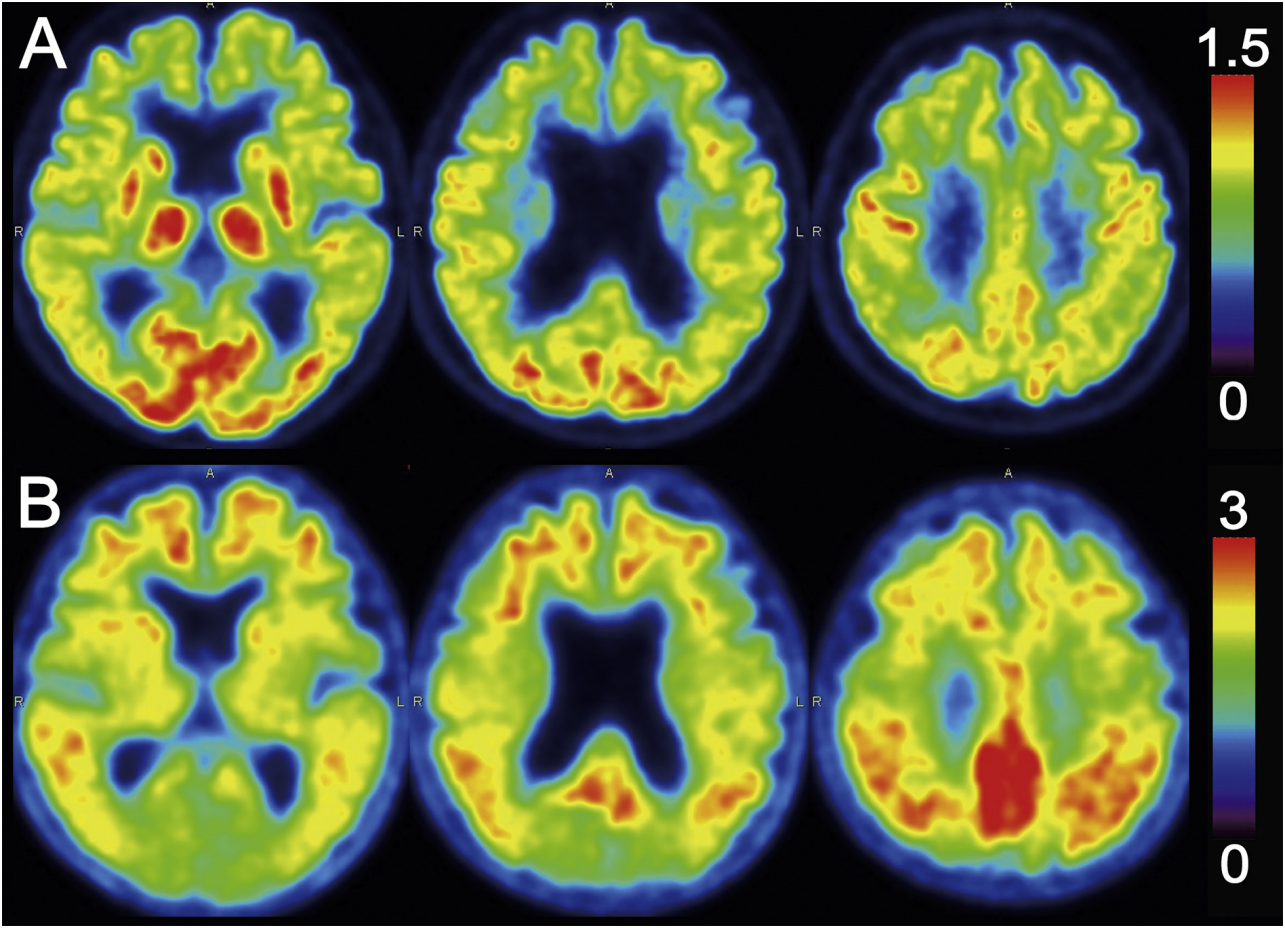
A representative case of ^18^F-FDG (A) and ^11^C-PiB (B) images. ^18^F-FDG and ^11^C-PiB images were normalized using the cerebellum as a reference region (*i.e.*, cerebellar uptake was set as one), and are displayed in axial sections. Especially in the posterior cingulate and precuneus cortices, ^18^F-FDG uptake decreased (A) and ^11^C-PiB uptake increased (B), showing a pattern characteristic of Alzheimer's disease. This case was from the patient 6 in Table 1.
